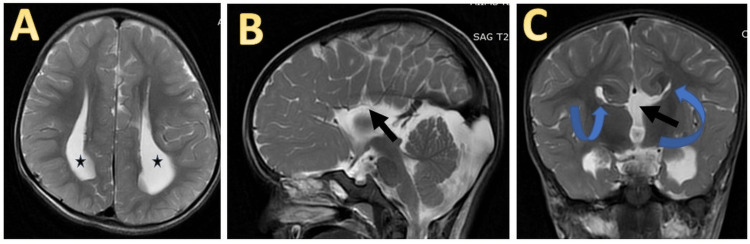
(A) T2-weighted MRI showing the widely separated body of the lateral ventricle with parallel orientation (*). (B) T2-weighted sagittal MRI showing a complete absence of the corpus callosum and cingulate sulcus with the hemispheric sulci seen reaching the third ventricle in a radial fashion giving a spoke wheel appearance (arrow). (C) T2-weighted coronal MRI showing a high-riding third ventricle (straight black arrow) communicating with interhemispheric cistern and the Viking helmet appearance of lateral ventricles (curved arrow).
